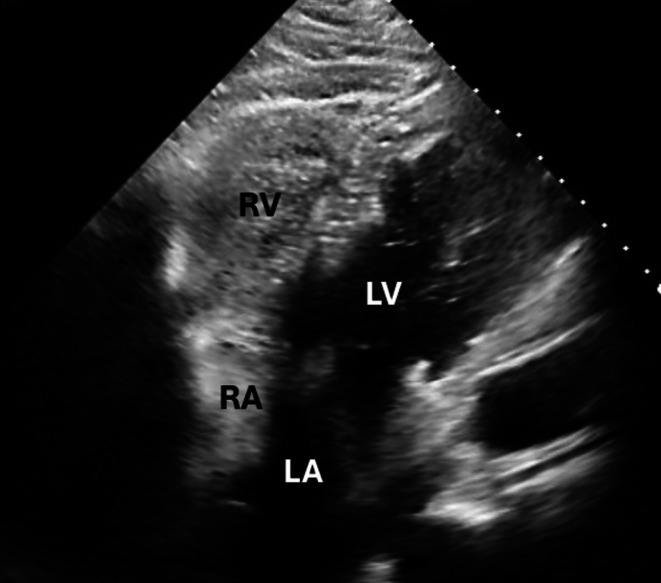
Transthoracic echocardiogram (TTE) with agitated saline showing right‐to‐left shunting. LA, left atrium; LV, left ventricle; RA, right atrium; RV, right ventricle.
